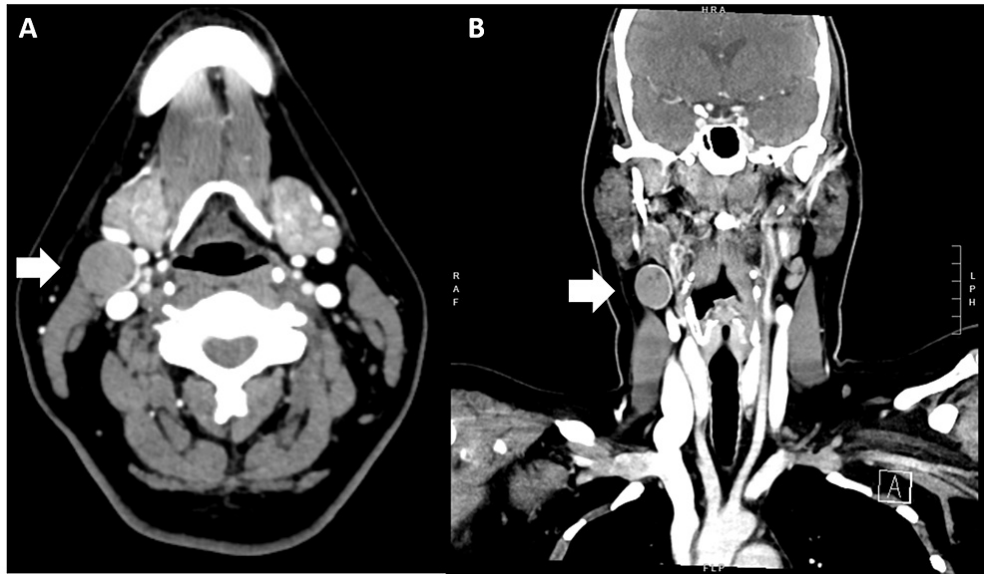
Computerized tomography (CT) scan of the neck with contrast (A) Axial and (B) coronal contrast-enhanced CT images reveal a 1.9 x 2.0 x 2.2 cm soft tissue lesion in the right neck compartment (level IIa) (white arrows) with peripheral enhancement and areas of central low attenuation. There is an associated nodular focus of hyperdensity that could represent enhancement versus calcification. This scan was obtained three months after the fine needle aspiration biopsy of the lesion.
